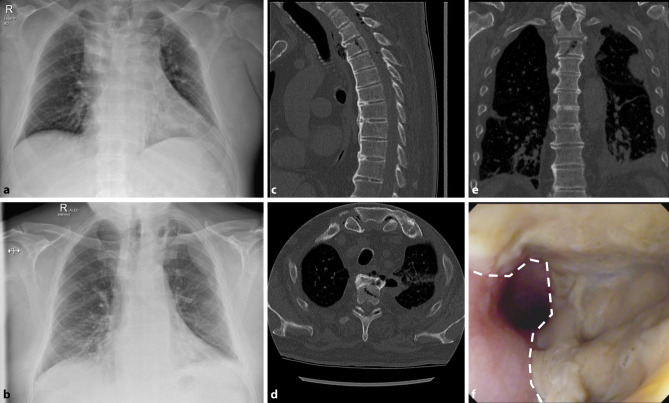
a At initial trauma the chest X-ray (frontal plane) taken in a lying position only showed the fracture of the 5th right rib. b Areas of encapsulated air and mediastinal widening were visible 5 days later on the chest X‑ray in standing position (frontal plane). Subsequent CT scan in sagittal (c), axial (d), and frontal (e) plane showed an incomplete burst fracture (c,d,e), esophageal perforation (d), mediastinitis with fistula (d), left-sided pleural empyema (d), and entrapped air within the spinal canal (c). Endoscopy prior to emergency esophagectomy revealed extensive necrosis involving two thirds of the esophageal wall (f; necrosis visible to the right of the dashed line)
